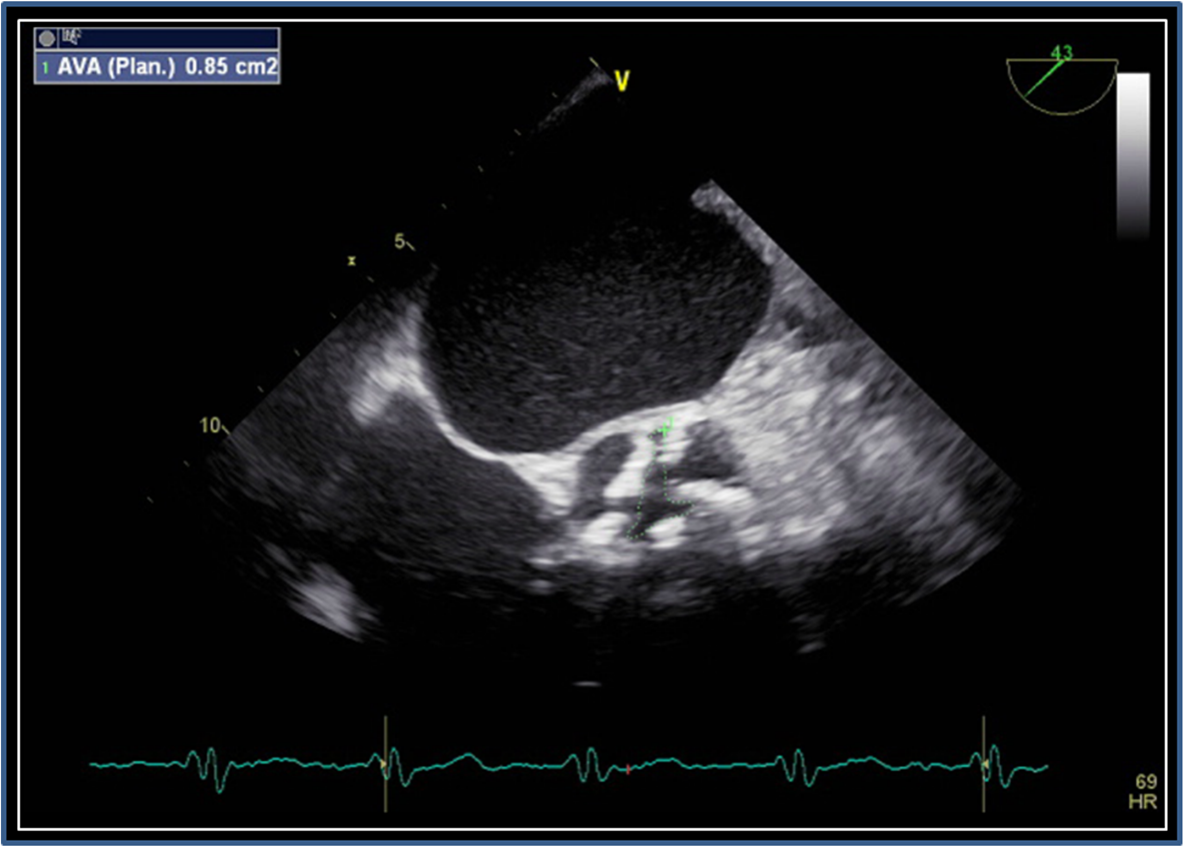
Morphologic aspect of the aortic valve in transesophageal echocardiography. Aortic valve area is calculated as 0.85 cm^2^ by planimetry
